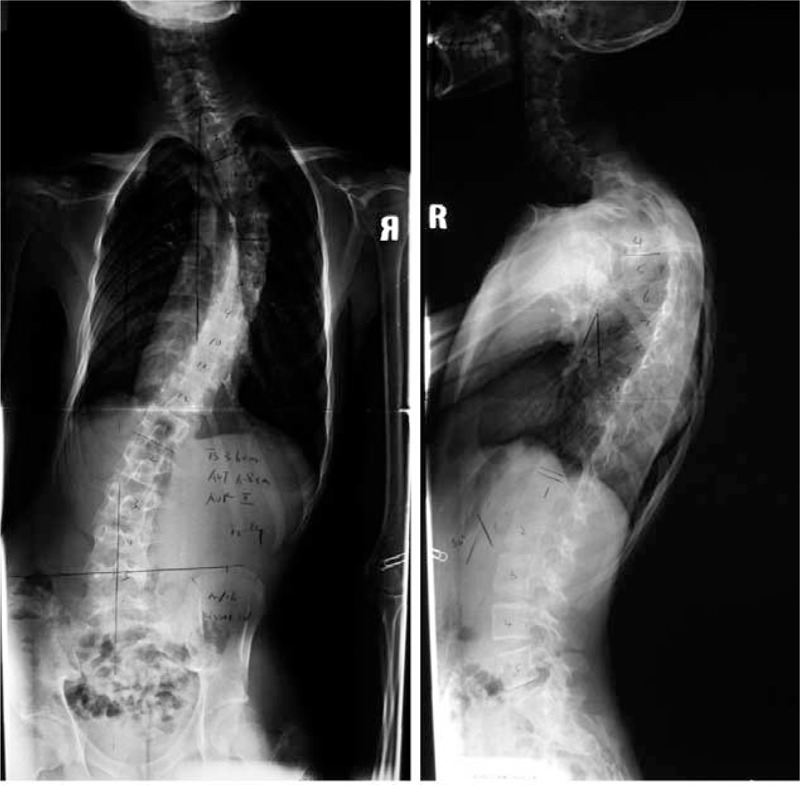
Standing anteroposterior and lateral radiographs of the preoperation on December 2009.
